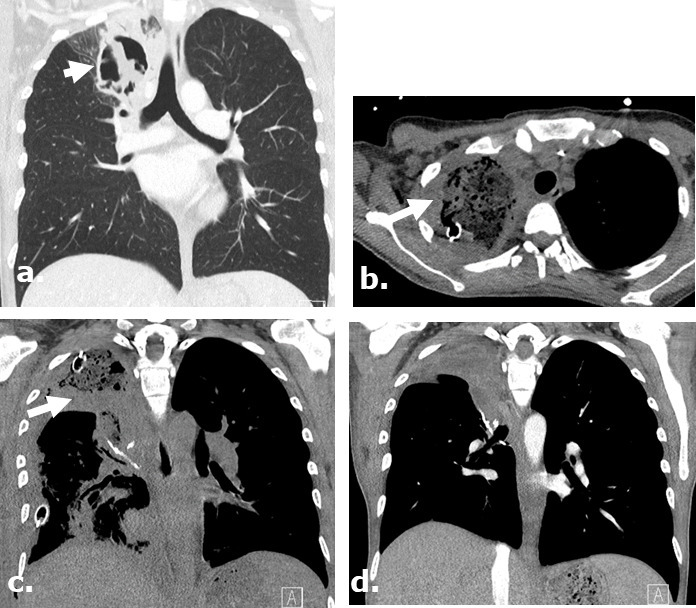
Surgicel in the post-operative bed mimicking infection following a lobectomy on CT. A 37-year-old female with AML and aspergilloma. Surgicel was used intraoperatively for hemostasis. Coronal (a) unenhanced CT images demonstrate an aspergilloma in the right upper lobe of the lung. Axial and coronal (b., (c) unenhanced CT images obtained 4 days post-surgery demonstrate large surgical cavity filled with low attenuation material mimicking a gas-containing infectious collection which was confirmed to be Surgicel on the operational note. Coronal (d) unenhanced CT images obtained 2 months after surgery demonstrate resolution of the finding.
